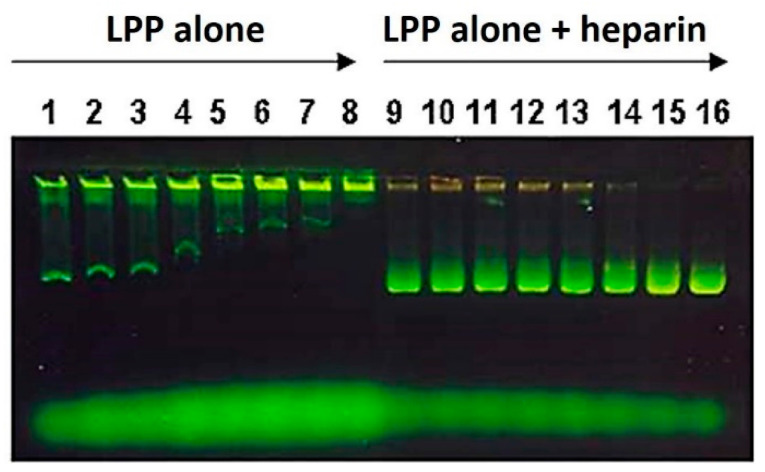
Determination of the optimal relationship between liposomes and polyplexes to form the lipopolyplex. The SUV SM: CH: DP lipopolyplex loaded with carboxyfluorescein was prepared at eight different mixing ratios by adding decreasing amounts of liposomes to a constant volume of the polyplex solution. On the left (lanes 1–8), samples of the lipopolyplex were loaded directly onto the gel. On the right (lanes 9–16), the samples of lipopolyplex were treated with heparin before loading on the gel.
